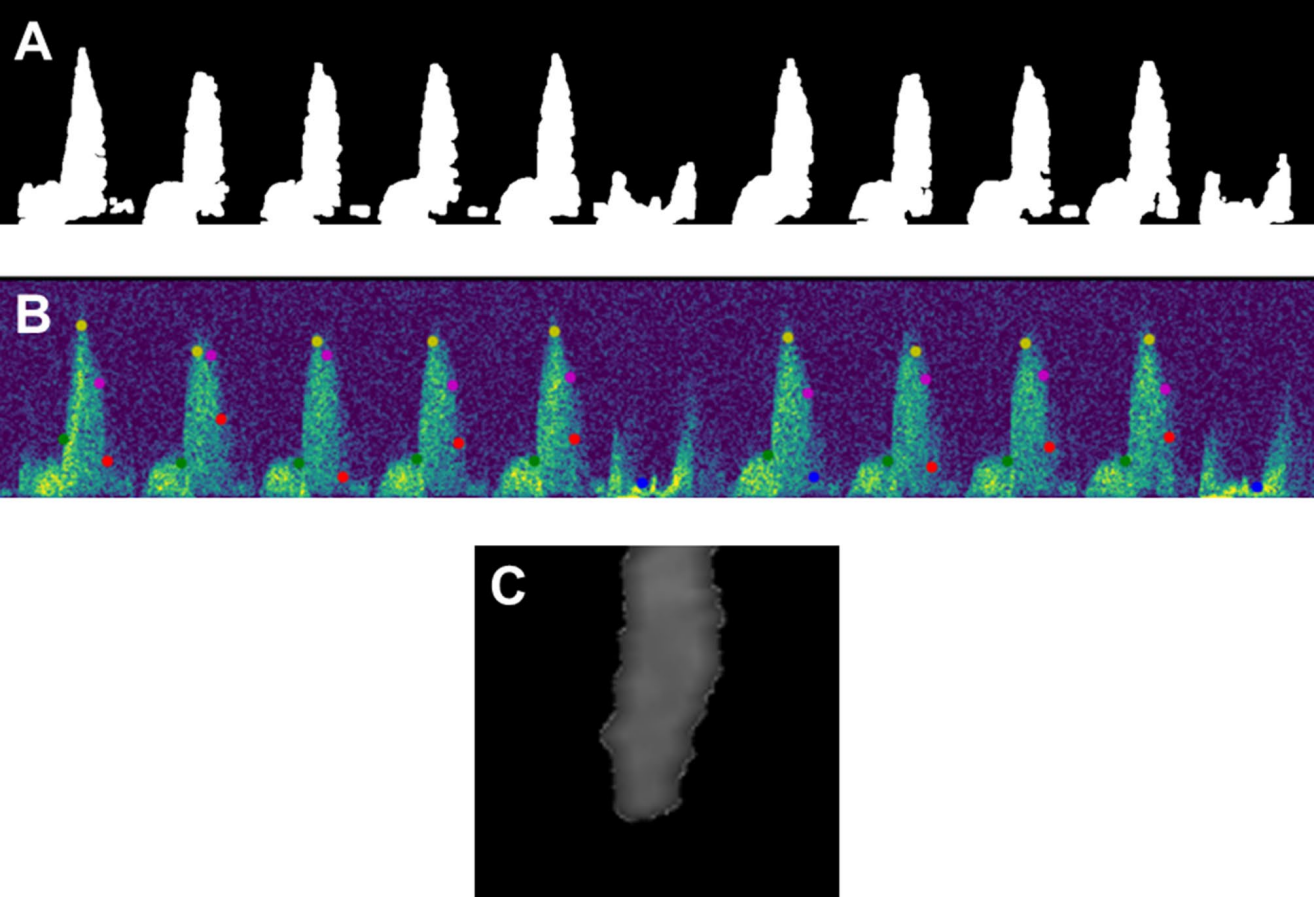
Images representing the steps taken by the Python algorithm for Doppler video analysis. Panel (A) displays a binarized image of the Doppler region, panel (B) shows the generated output image with critical values labeled with colored points, and panel (C) is an example of a vessel diameter measured during Color Mode analysis.
